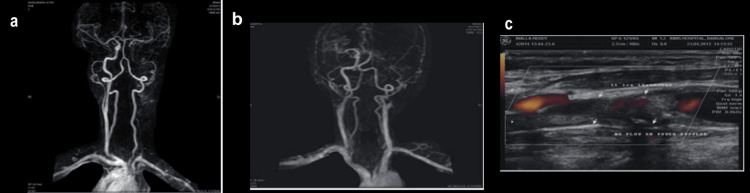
Imaging findings demonstrating carotid artery stenosis and occlusion a, b: magnetic resonance angiography (MRA) of the neck vessels showing complete occlusion of the left internal carotid artery (ICA) with absence of flow-related enhancement distal to the carotid bifurcation, collateral flow through the circle of Willis is visualized; c: the Color Doppler ultrasound image demonstrates the absence of flow in the left ICA in power Doppler mode, confirming complete occlusion, the lumen appears echogenic and filled with thrombotic material (arrows), consistent with a chronic occlusive plaque.
